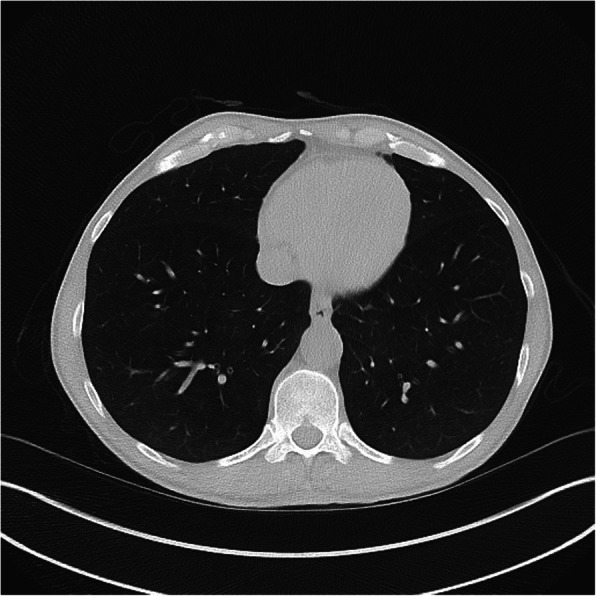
Follow up chest CT showing complete resolution of the ground glass opacities
